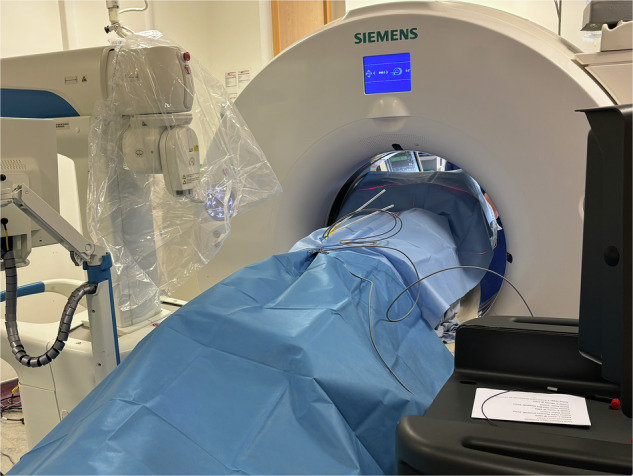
Procedural setup. Procedural setup for robot-assisted CT-guided lung cryoablation showing the robotic arm (left, plastic-covered), CT gantry, and immobilised, draped patient under general anaesthesia with high-frequency jet ventilation. Cryoprobes and cabling are also visible
